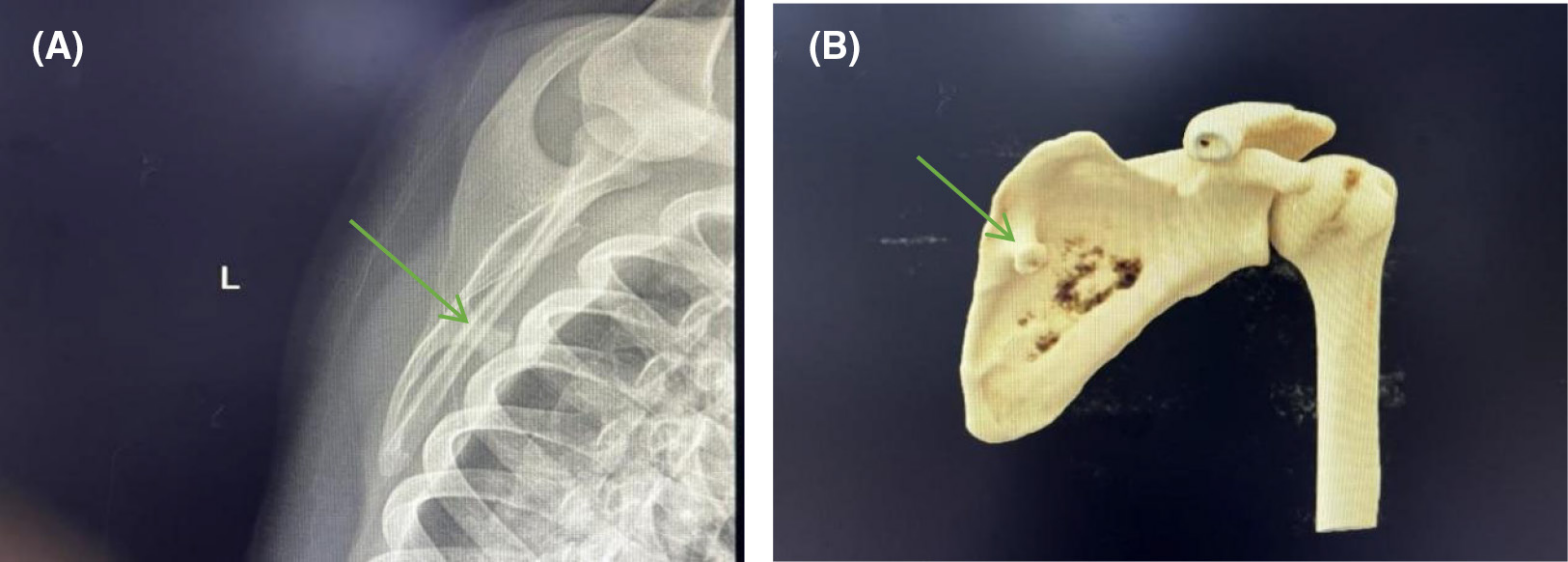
(A) Radiograph showing a pedunculated bony mass extruding from the left scapula ventral surface. (B) Computed tomography (CT) scanner 3D reconstruction showing a bony exostosis near the medial border on the ventral of the left scapula.
